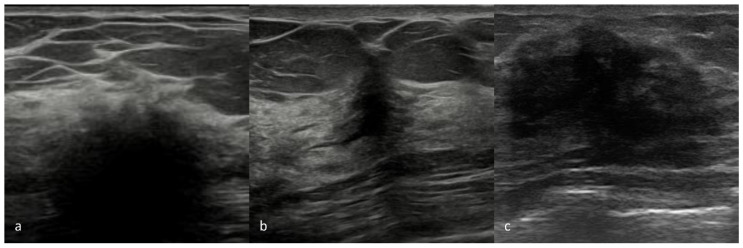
B-mode ultrasound images of a 34-year-old female with DM1, Hashimoto’s disease, and a DMP diagnosis following core needle biopsy show inhomogeneous hypoechogenic areas with irregular margins (a) and irregular hypoechogenic areas with indistinct margins, a non-parallel orientation, and posterior acoustic shadowing (b), indicating DMP lesions. (c) Inhomogeneous hypoechogenic areas with irregular margins of a 39-year-old woman with DM1 and an invasive ductal carcinoma diagnosis who underwent core needle biopsy.
